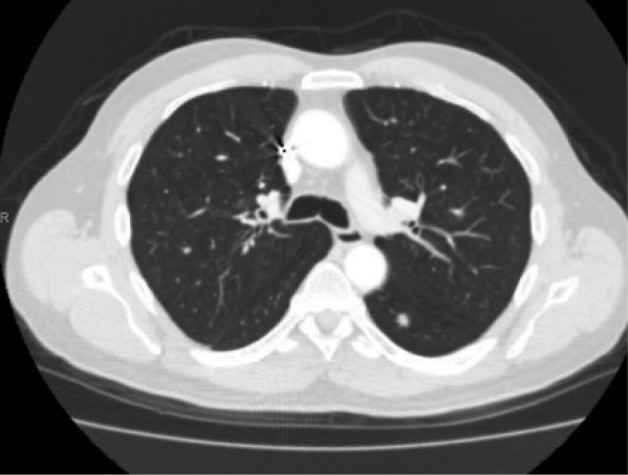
September 2011 CT scan of the chest, abdomen, and pelvis reveals a 7-mm left lower-lobe pulmonary nodule.
